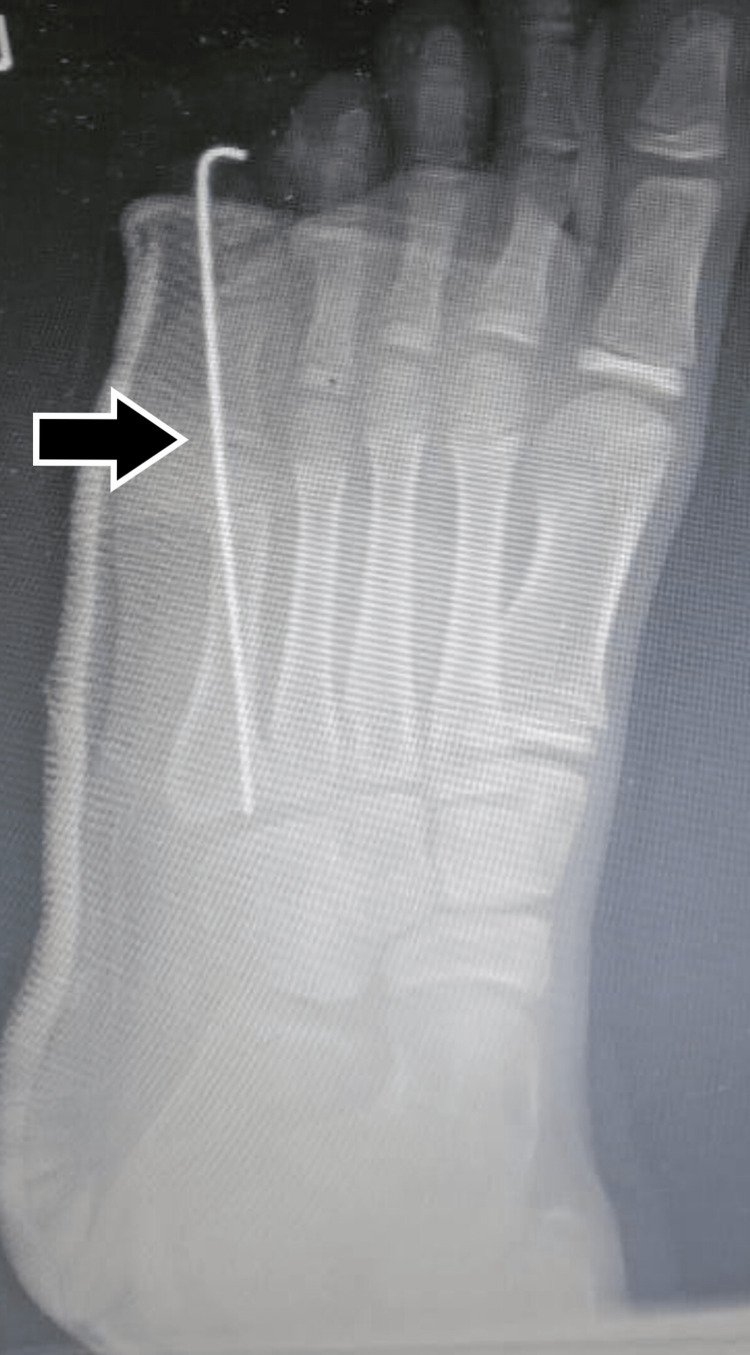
Immediate post-operative x-ray with k-wire
